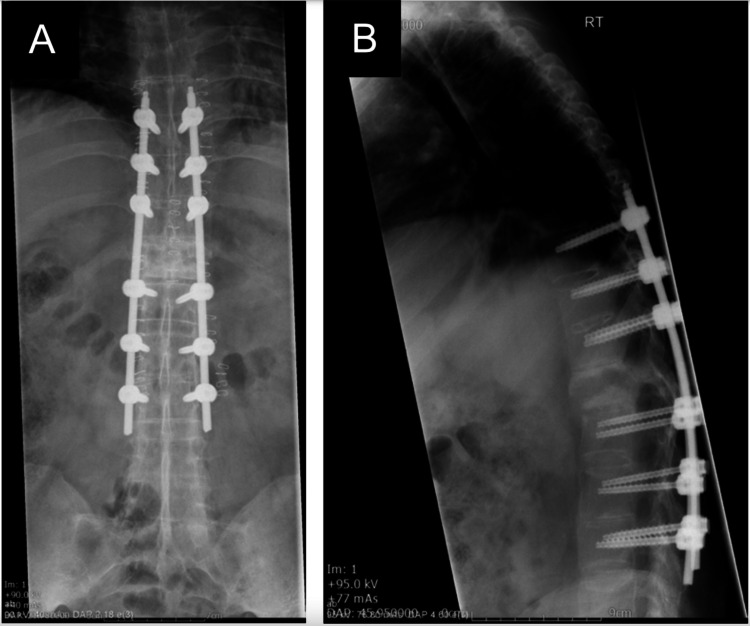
Post-operative radiographs (A) anterior-posterior view, (B) lateral view demonstrating good fracture reduction and satisfactory metalwork placement.
